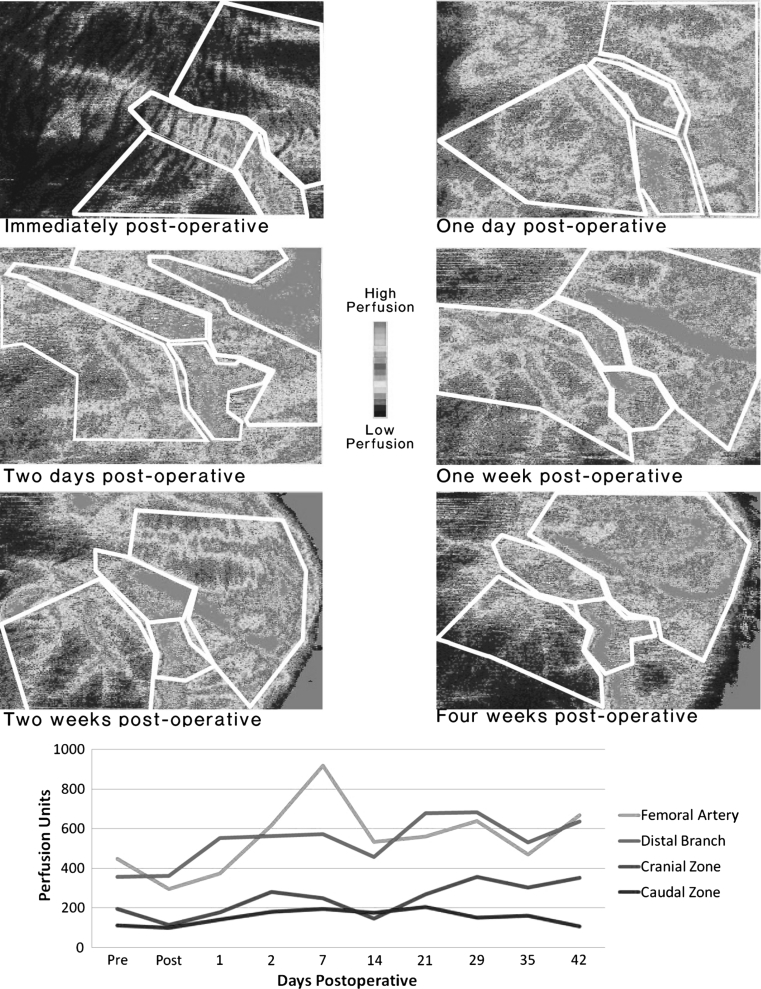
Laser Doppler scans at successive time points in a typical subject (*above*) and the corresponding plot of mean perfusion by zones
